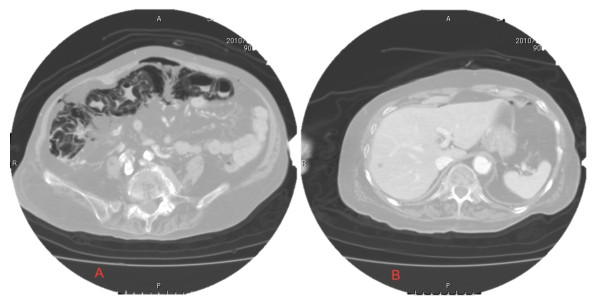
CT. Abdominal CT reveals diffuse intramural gas from the ascending colon to the transverse colon and a large amount of free air in the abdominal cavity without portal venous air or extraluminal fluid collections. This study shows diffuse pneumoperitoneum, which led us to suspect the presence of gastrointestinal perforation. Portal venous gas, which frequently follows severe pneumatosis intestinalis, is also absent.
